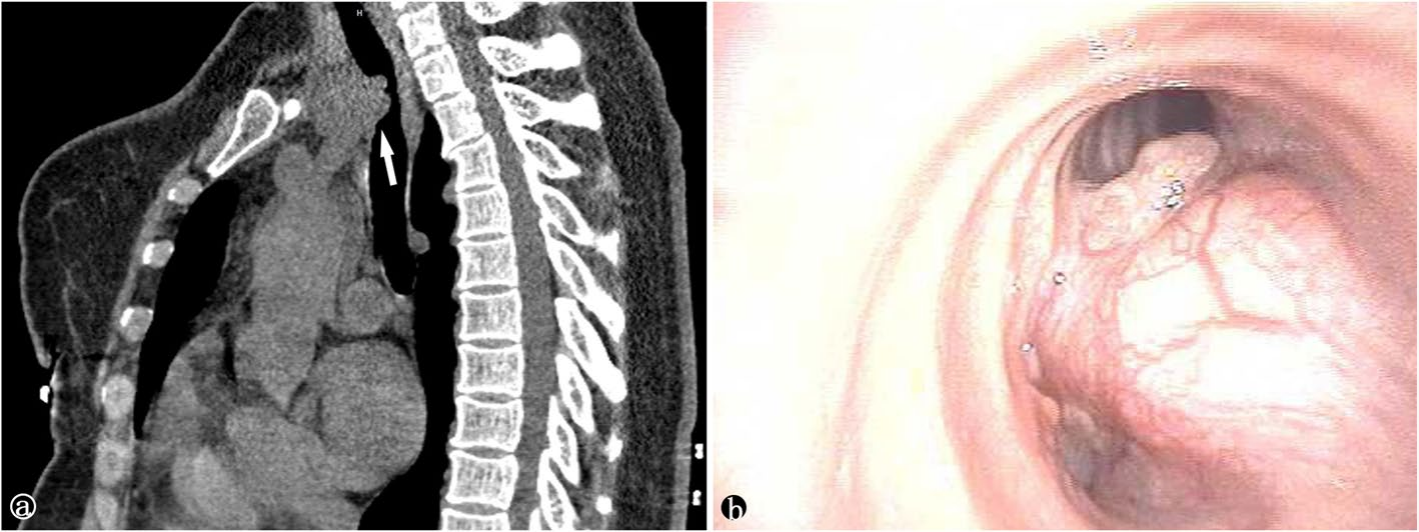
a CT scan of neck revealed a mass attached to the tracheal. b Adenoid cystic carcinoma almost complete obliteration of the lumen of the bronchus
